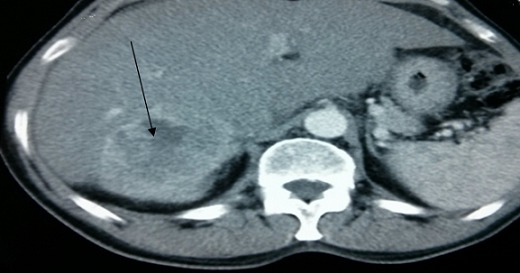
CT scan image of a PHNEC patient; CT scan showing a liver mass (segment VI and VII)
